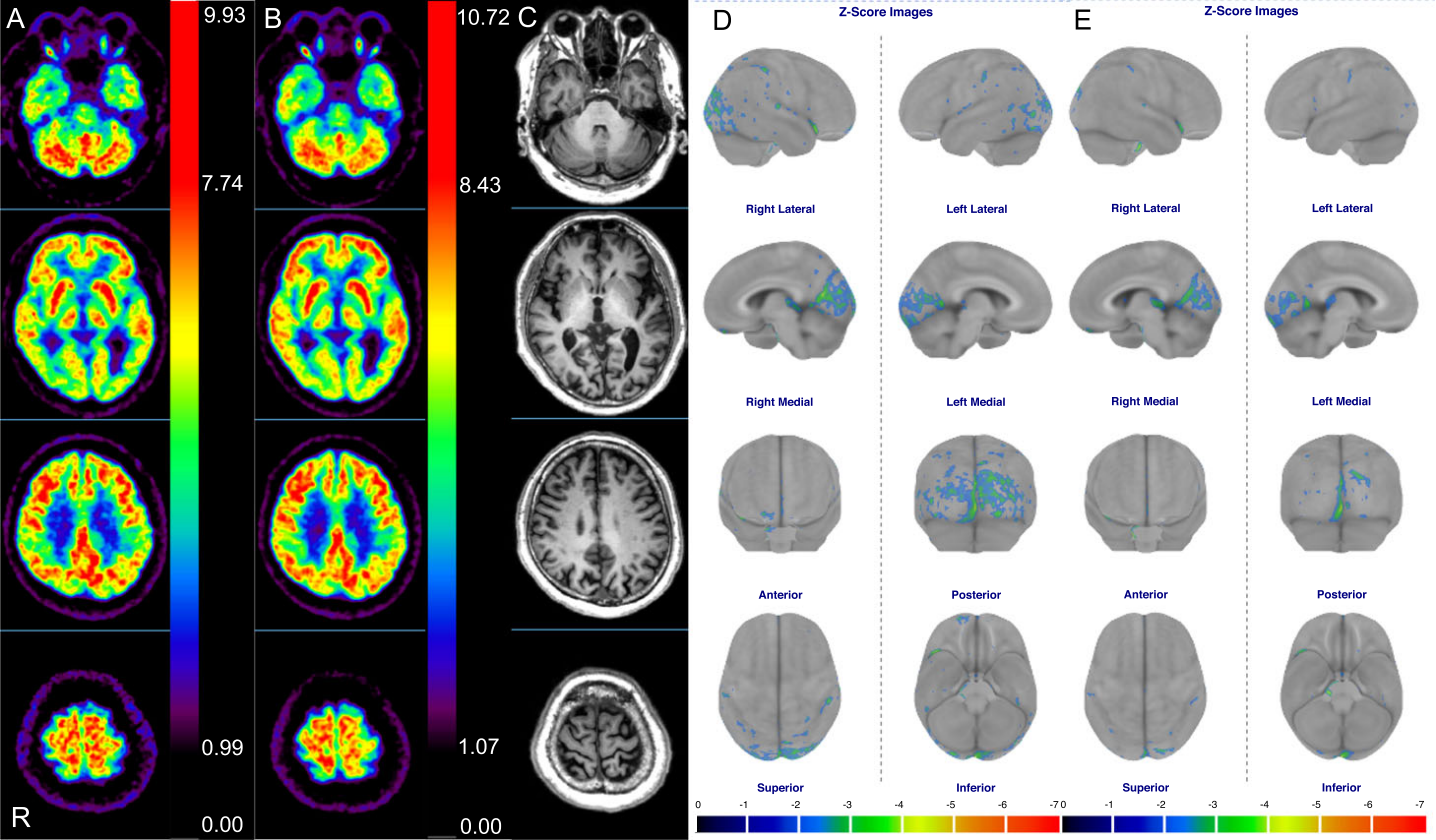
[^18^F]FDG images, MR images, and *Z*-score maps derived from a 71-year-old male. [^18^F]FDG images acquired using PMT-PET (a) and SiPM-PET (b). MR image of slices of both [^18^F]FDG images (c). *Z*-score maps calculated from PMT-PET (d) and SiPM-PET (e) images. MR magnetic resonance, PET positron emission tomography, PMT photomultiplier tube, SiPM silicon photomultiplier
